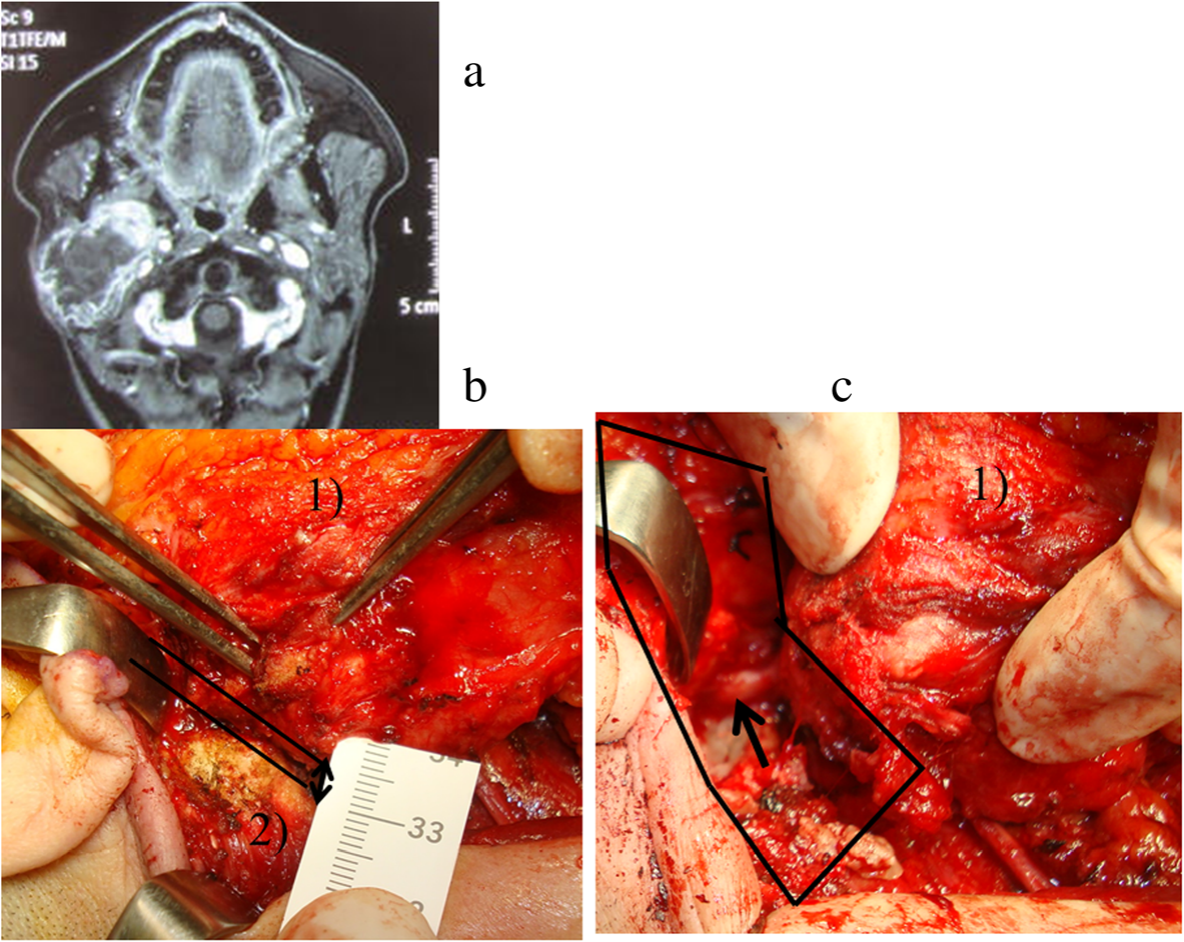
Recurrent parotid cancer. a). MRI. b). Intraoperative findings ( before removing mastoid process). Working space is significantly narrow (↔) between mastoid process and the tumor. 1) the tumor, 2) mastoid process. c). After removing mastoid process. Expanding the working space facilitates identification of the facial nerve and dissection of parotid tumor without retracting the facial nerve (arrow).
